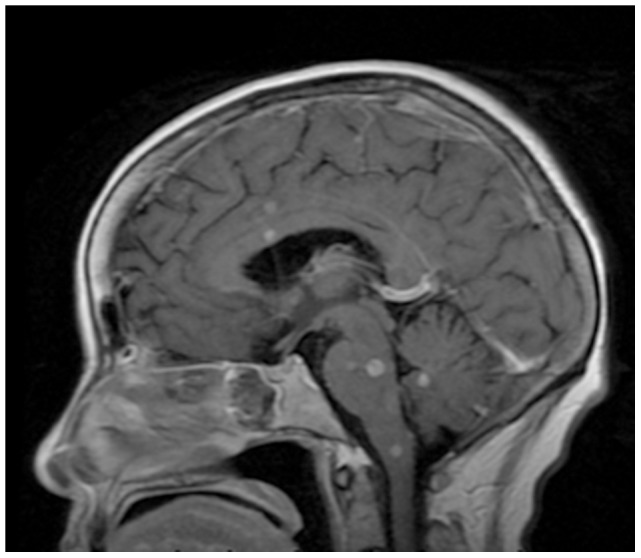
Enhanced MRI scan shows tuberculous, ring-shaped, signal-intensified lesions in bilateral frontal and parietal lobes, centrum semiovale, peri-lateral ventricular region, basal ganglia regions, thalamus, brainstem and cerebellum.
